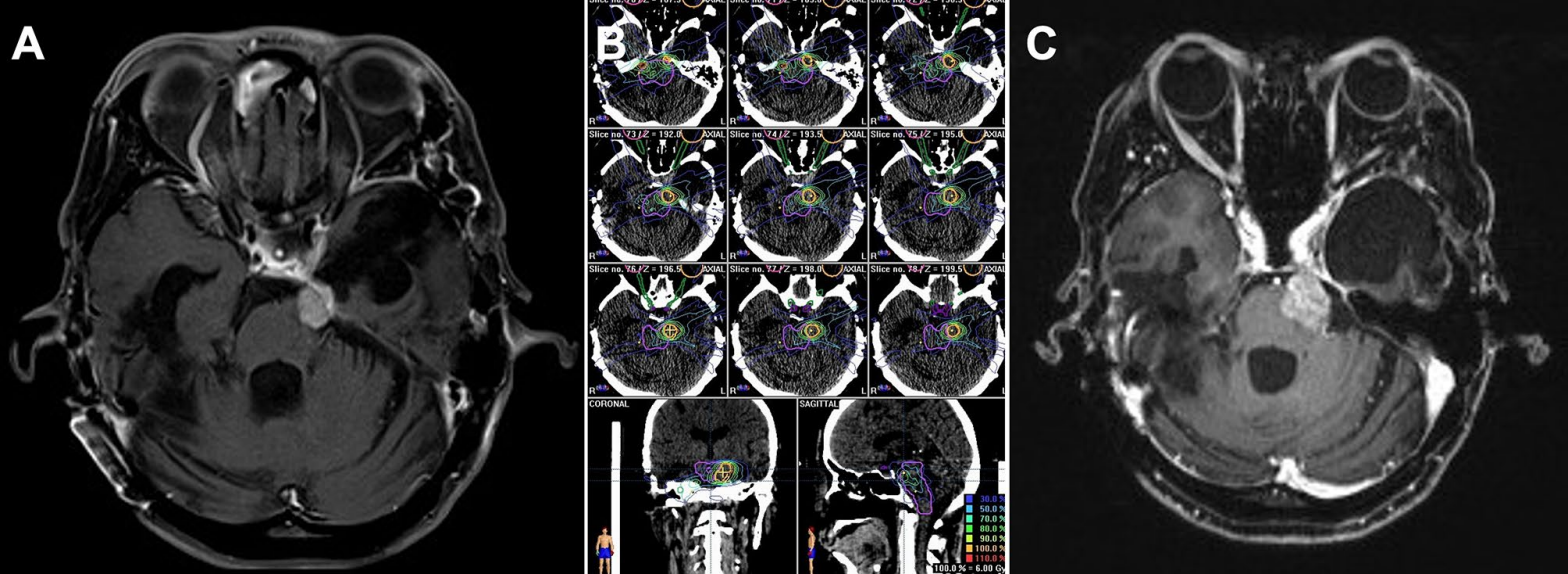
A 47-year-old female with a cerebellopontine angle meningioma (volume, 2.69 ml). Treatment was 21 Gy in three fractions. (A) Pre-treatment magnetic resonance imaging (MRI) scan. (B) Treatment plan showing the resulting isodose line. (B) MRI scan 98-months after treatment showing tumor progression.
